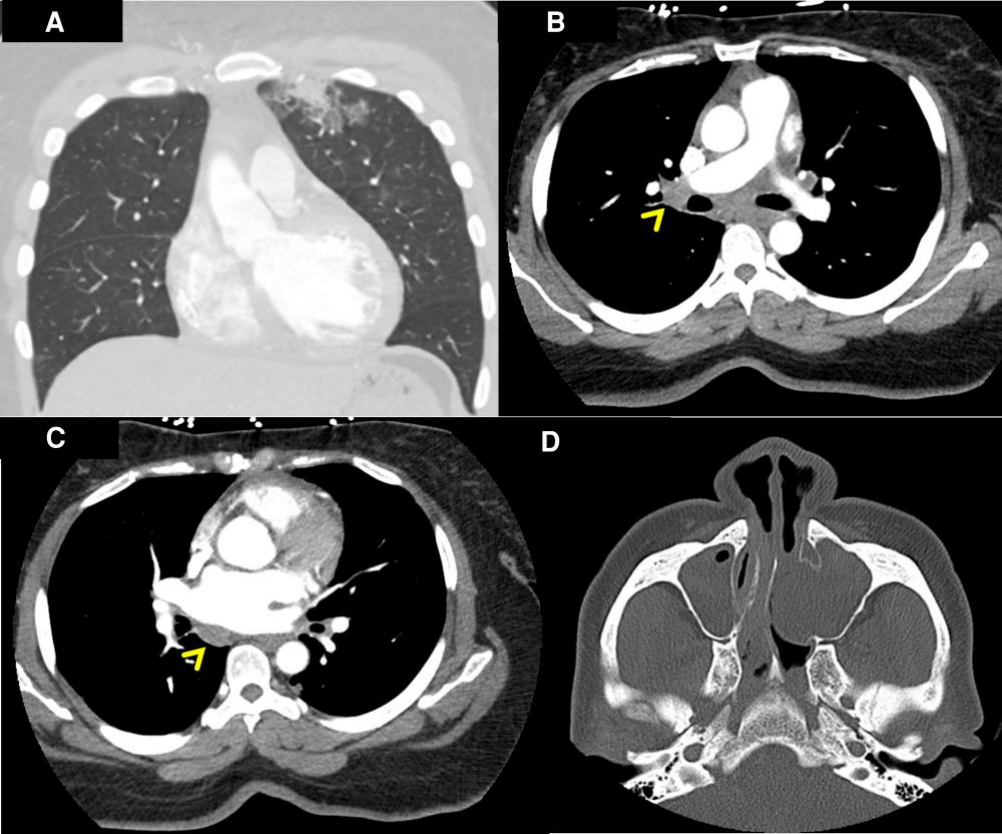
Computed tomography of the chest and sinuses. Patchy bilateral ground glass and airspace opacities were noted in the left upper lobe on computed tomography of the chest with intravenous contrast (*A*). Mediastinal lymphadenopathies were also visualized (linear arrowheads in *B* and *C*). Left antrochoanal polyp was found on computed tomography of the sinuses with intravenous contrast (*D*).
